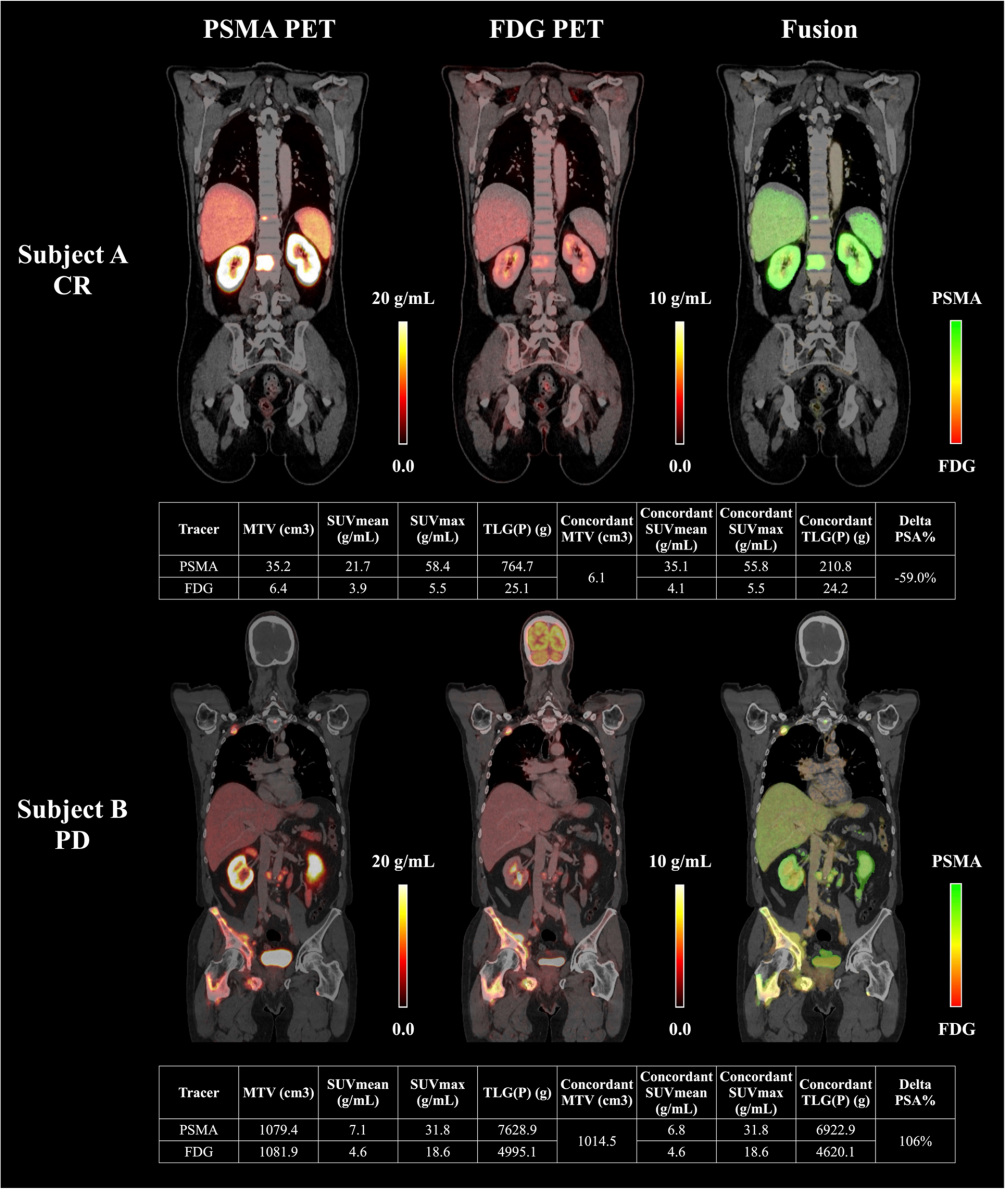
representative examples of dual-tracer pet/ct imaging and concordant lesion analysis in responders and non-responders to psma-rlt. Quantitative imaging biomarkers of concordant and non-concordant lesions, including molecular tumor volume (MTV), SUV_mean_, SUV_max_, total lesion PSMA (TLP), total lesion glycolysis (TLG), are summarized in the tables for both tracers. Coronal slices of PSMA PET, FDG PET, and fused PSMA/FDG PET (PSMA uptake = green, FDG uptake = red, Concordance = yellow) are shown for two patients: Subject A (Gleason score 10, status post androgen deprivation therapy (ADT) and chemotherapy, PSA of 9.9 µg/L at the time of imaging) exhibited metastatic involvement in bone (thoracic and lumbar vertebrae), in retroperitoneal lymph nodes, and in two intraprostatic lesions, with only low concordant tumor burden. This patient showed a complete PSA response (CR, ΔPSA = –59.0%); Subject B (Gleason score 8, status post radiotherapy to the prostate, ADT, androgen receptor pathway inhibitor and chemotherapy, PSA of 109 µg/L at the time of imaging) exhibited widespread metastases, including multiple bone lesions (upper and lower skeletal) and retroperitoneal lymph nodes, with extensive concordant tumor volume. This patient demonstrated progressive disease (PD, ΔPSA = + 106%)
